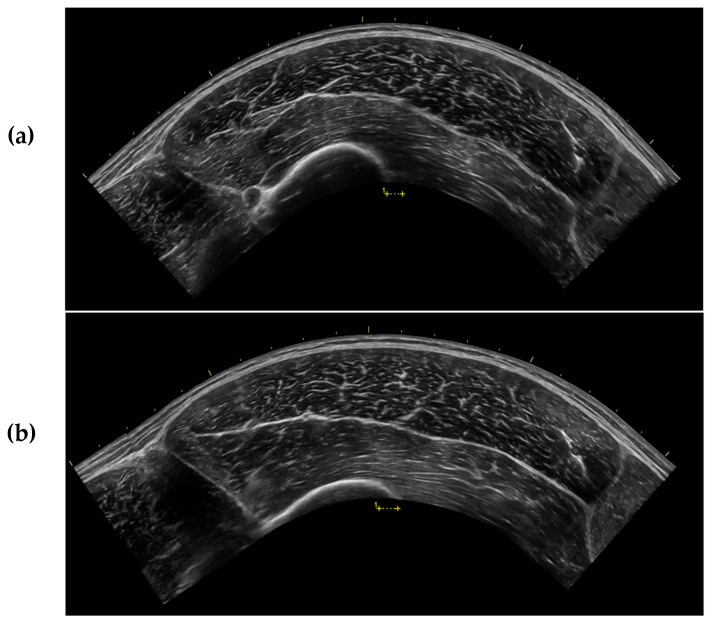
Sample ultrasound images captured from the same participant after 15 min of rest in (a) the supine position (SUP) and (b) during standing (ST). All ultrasound settings were kept consistent throughout testing for each participant.
